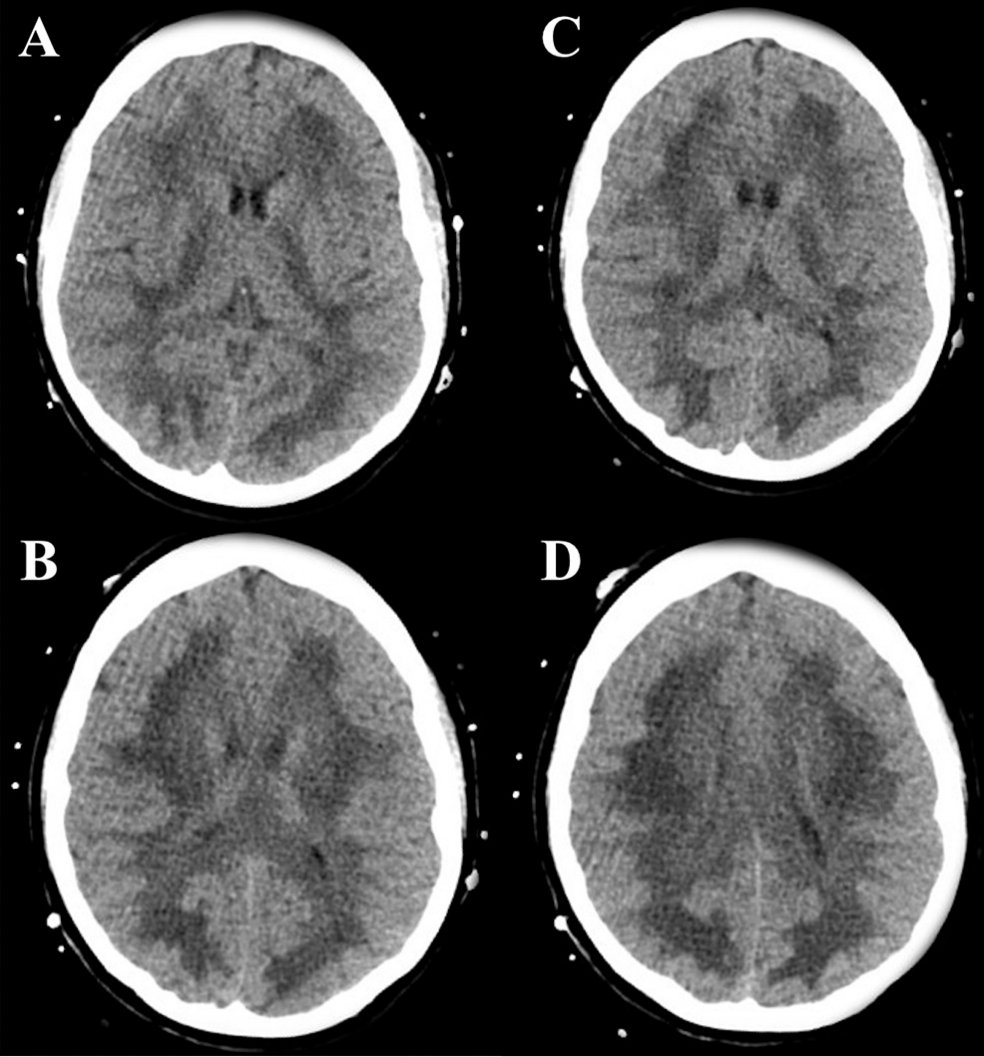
Representative images of the CT head without contrast obtained on admission. There is diffuse symmetric hypodensity throughout the white matter, more pronounced at the corona radiata, without involvement of the gray matter. Panels are arranged from most caudal (A) to most cranial (D).
